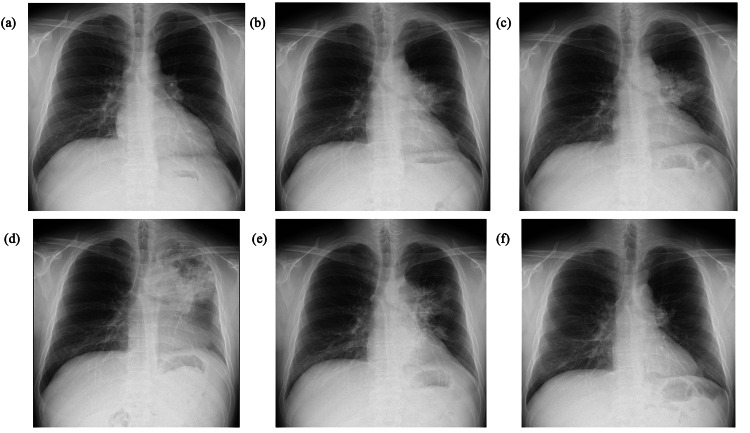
The treatment course and chest radiography findings of the patient. (a) Chest radiography at the first visit indicated mediastinal lymphadenopathy. (b) On day 188, a new infiltrative shadow was evident in the left upper lung field. (c) On day 199, the infiltrative shadow in the left upper lung field had worsened. (d) On day 227, the infiltrative shadow showed further progression. (e) On day 406, the infiltrative shadow showed signs of improvement. (f) Observations on day 761 indicated that the infiltrative shadow had resolved, but mild mediastinal lymph node swelling remained.
